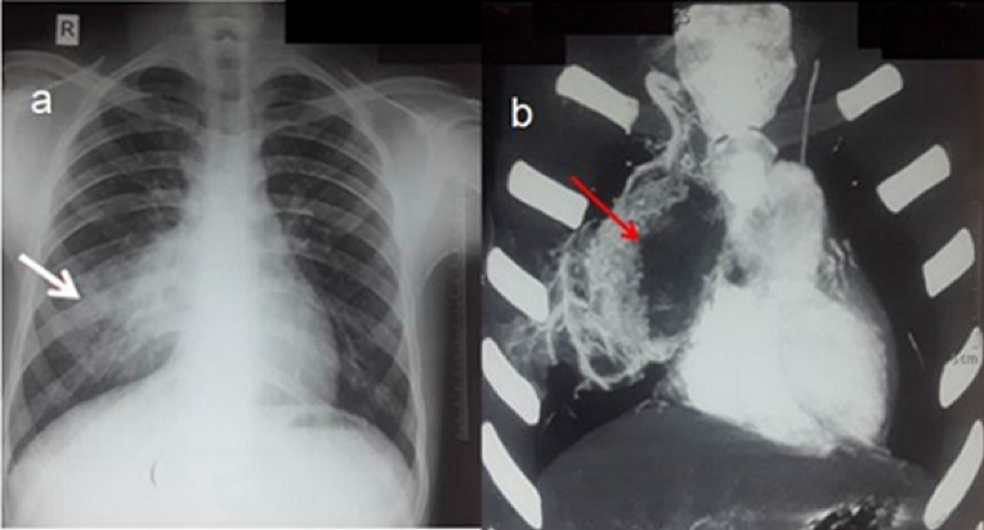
(a) Chest X-ray showing an ill-defined mass in the right hilar region. (arrow showing perihilar mass). (b) Contrast-enhanced CT of thorax showing an 8x8 cm heterogeneous mass in anterior mediastinum compressing the right atrium and abutting the right ventricle (arrow showing mediastinal mass).
